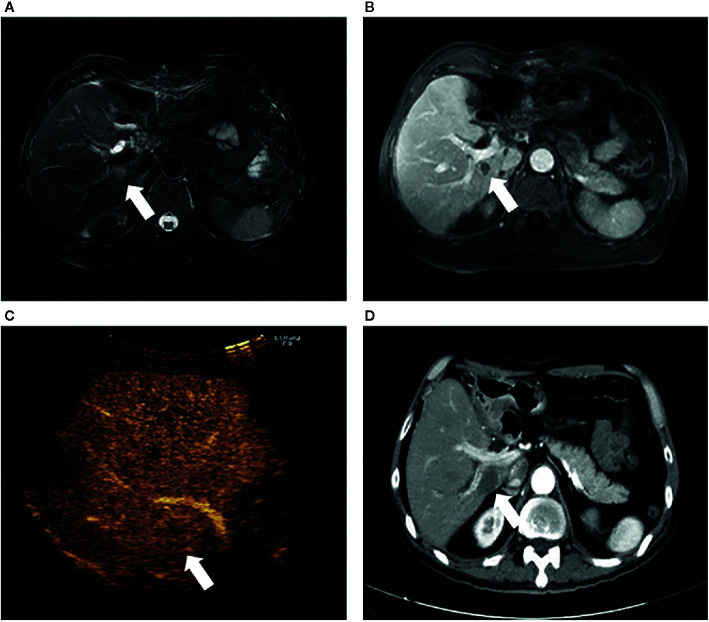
Images in a 61-year-old-man who underwent radiofrequency ablation (RFA) for periportal CLM. (A) Axial MRI T2-weighted images shows a 2.2-cm lesion of high signal intensity (arrow) in segment VII before RFA. (B) Axial enhanced MRI image shows that the lesion (arrow) washes out in equilibrium phase; (C) contrast-enhanced ultrasonography (CEUS) image before RFA shows that the index tumor (arrow) is in contact with the portal vein. The patient underwent RFA, and obtained technical effectiveness 1 month after RFA. (D) Axial enhanced CT image shows no local tumor progression around the ablation zone 17 months after RFA. CLM, colorectal liver metastases; US, ultrasound; RFA, radiofrequency ablation.
